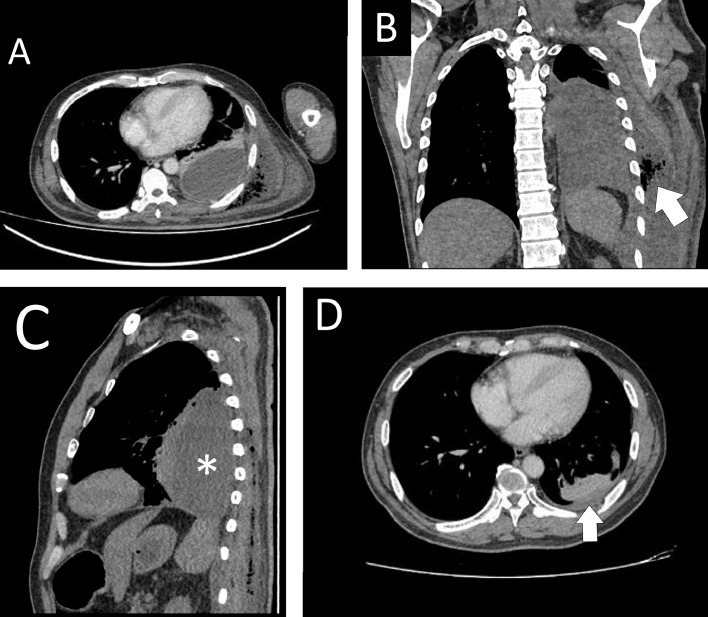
(A) Enhanced-contrast CT of the thorax at the axial view, showing a hypodensity in the left pleural cavity (*) with an extensive subcutaneous, extrathoracic, gas-forming extension (white arrow) of the collection to the left posterior chest wall, suggestive of left ET. (B) The collection with gas-forming extension (white arrow) at the coronal view. (C) The collection (*) at sagittal view. (D) A repeat CT of the thorax, revealing a reduction of the left pleural effusion (white arrow) and left posterior chest wall collection after completing 2 weeks of antibiotics.
